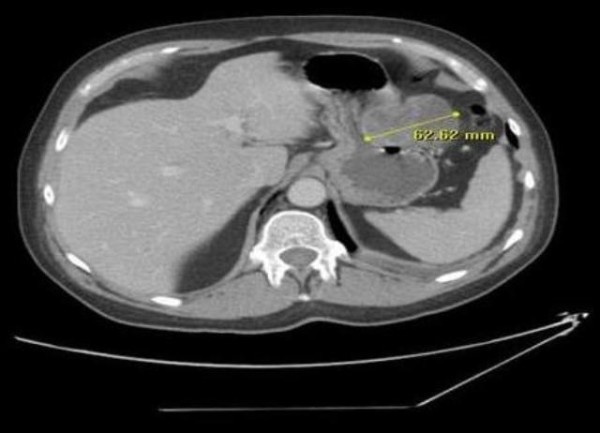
CT scan revealing 6 cm mass with no evidence of extraperitoneal spread.
